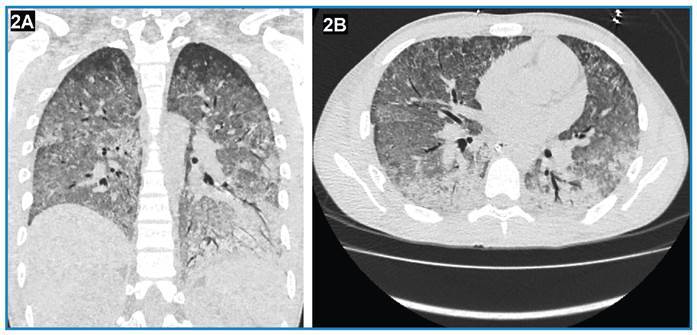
High-resolution chest computed tomography (coronal (A) and transverse (B) planes) showing alteration in the attenuation coefficients of the lung parenchyma due to diffuse ground-glass attenuation of the parenchyma of both lungs, with thickening of the interlobular and intralobular septa and bilateral consolidation in the lower lobes, primarily in the basal segments.
